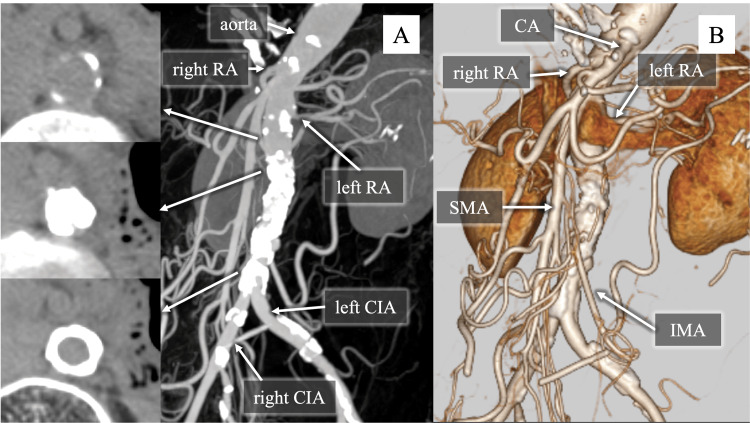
Preoperative CT images (A) The plain CT image reveals extensive calcification of the infrarenal aorta and localized stenotic lesions, whereas only mild calcification is noted in the aorta just below left RA bifurcation and in both common iliac arteries. (B) The contrast-enhanced CT image shows that the CA, SMA, IMA, and both right and left renal arteries are patent, with no significant stenosis.
